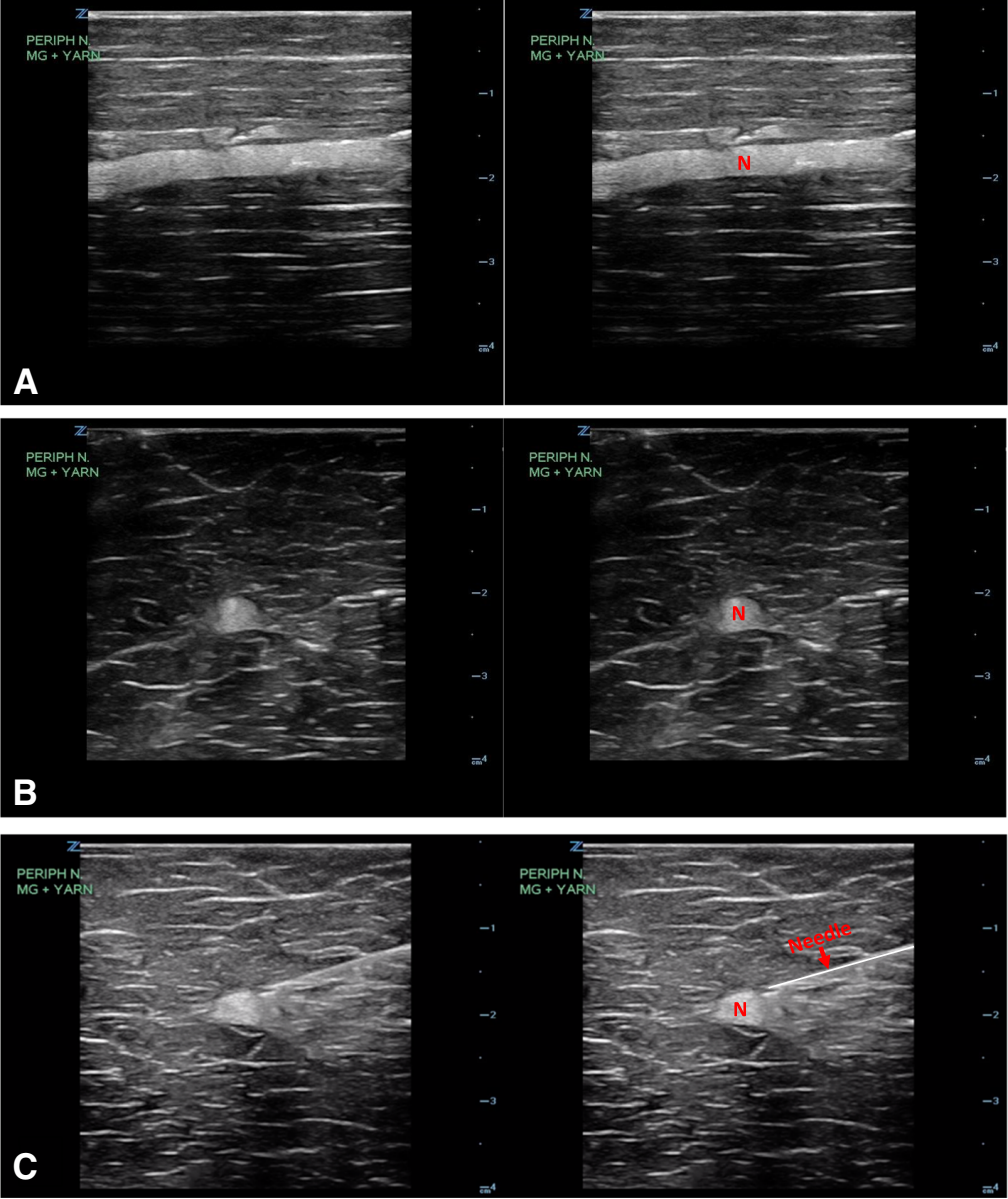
Ultrasound image of the peripheral nerve model in longitudinal (a) and transverse views (b). Ultrasound image of the transverse view with the needle placement (c). N, nerve
